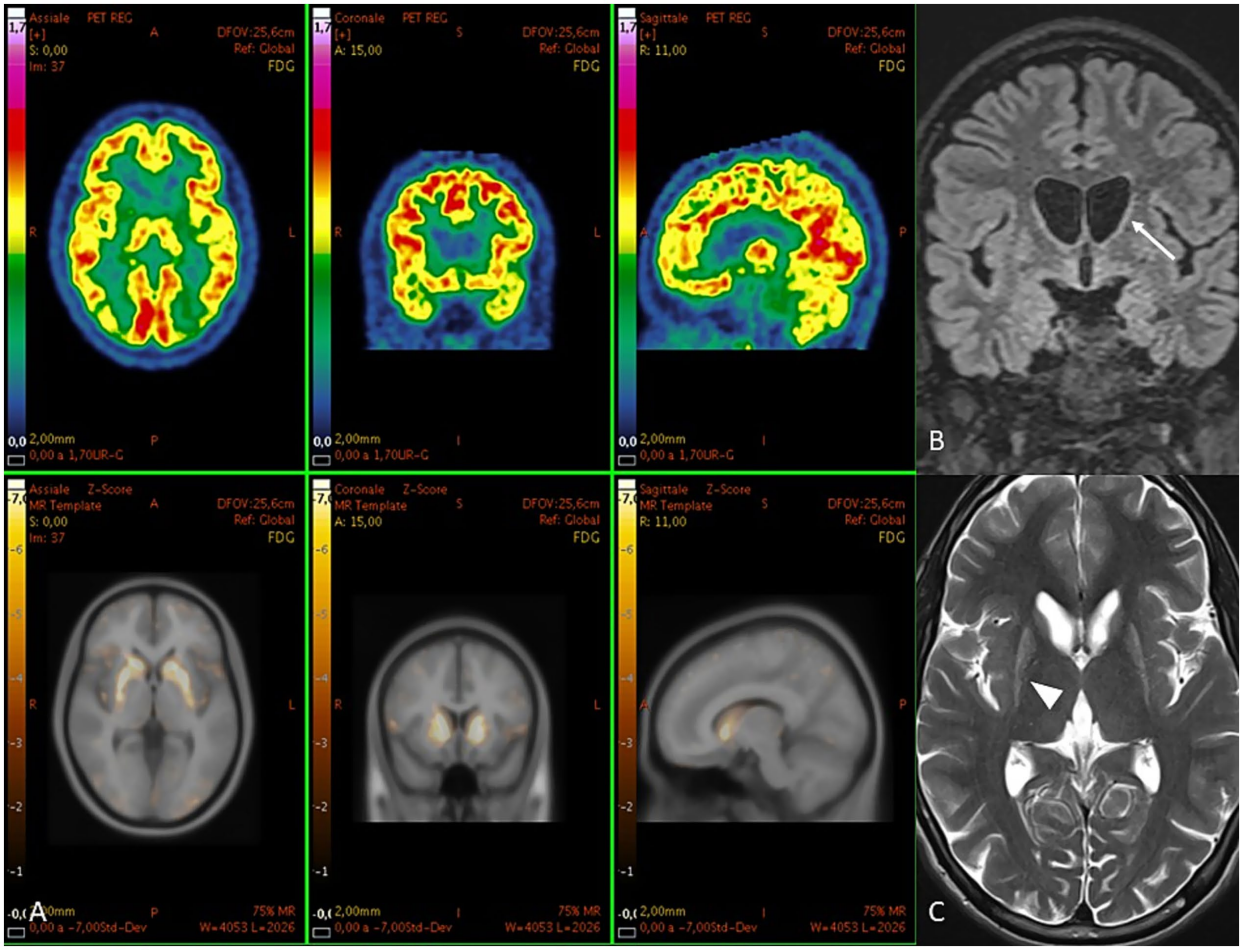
(A) PET images in patient two. Above [18F] FDG images and below their *z*-score maps show reduced uptake in caudates and putamina. At MRI, (B) coronal FLAIR shows a symmetric flattening of the lateral profile of the frontal horn due to atrophy of the caudate head (arrow). (C) axial T2 shows hyperintense and atrophic putamina (arrowhead).
